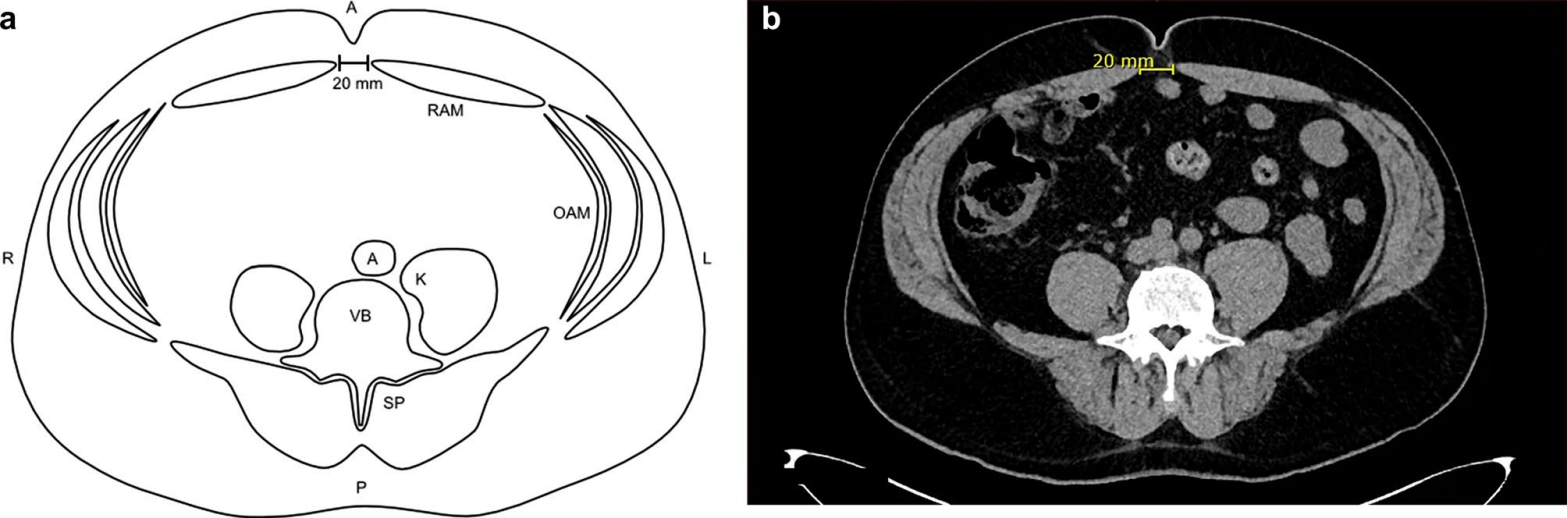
a, b Measurement of the IRD showed 20 mm at the umbilicus on a CT scan^5^. ^5^*A* aorta, *K* kidney, *OAM* oblique abdominal muscles, *RAM* rectus abdominis muscle, *SE* spinal erectors, *VB* vertebral body
